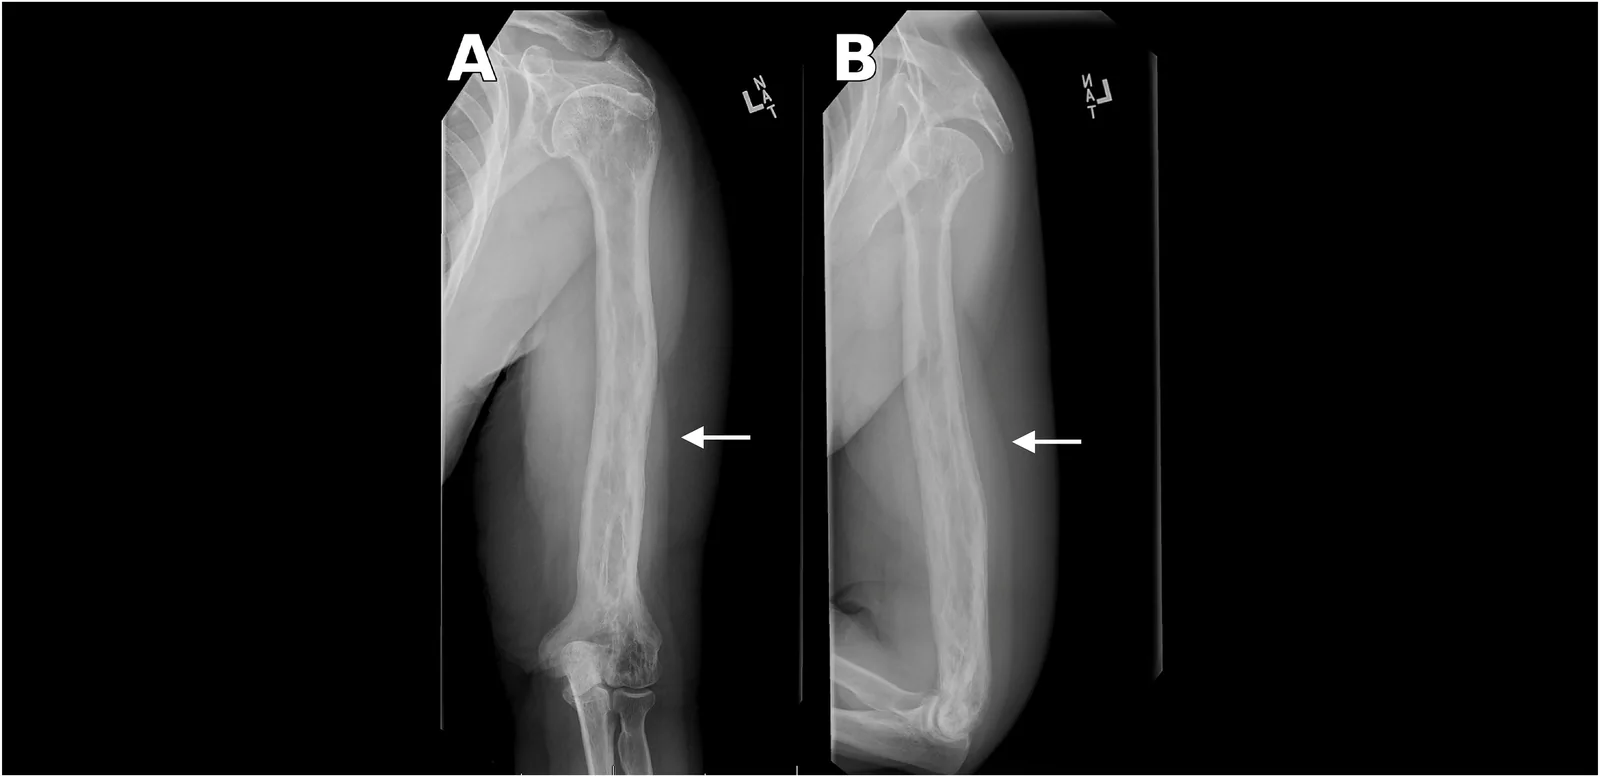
(A) Anteroposterior and (B) lateral radiographs of the left humerus demonstrating mild curvature (arrows) of the diaphysis, convex laterally, with cortical and trabecular thickening with increased width in the mid and distal diaphysis (bracket). Created in BioRender.com by JJL.
